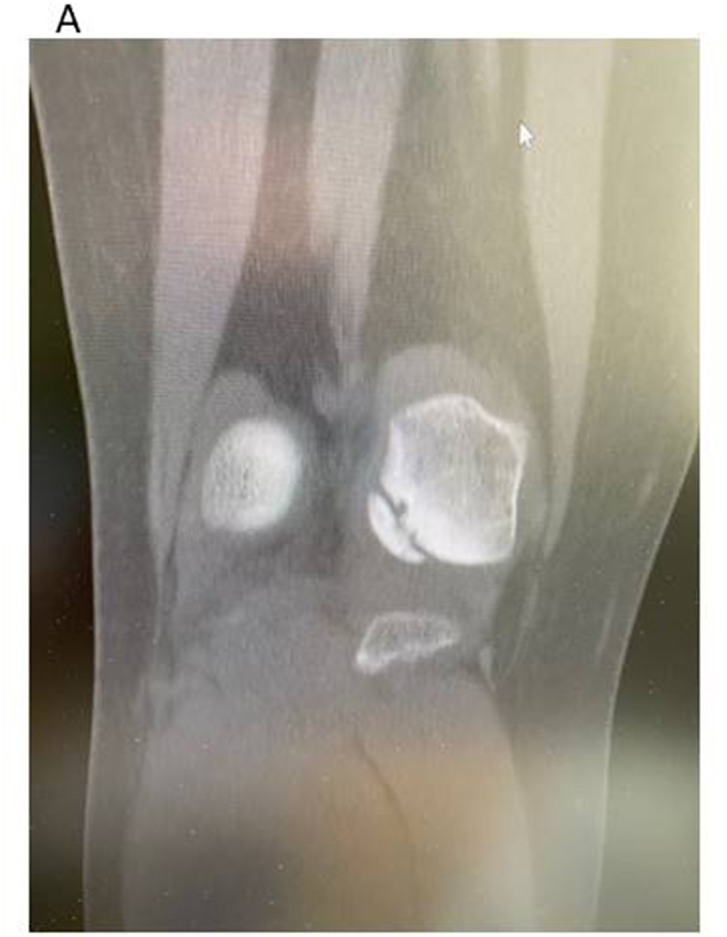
A medial femoral condyle lesion seen on CT scan image. It is obvious that it is loose with no bony contact.
